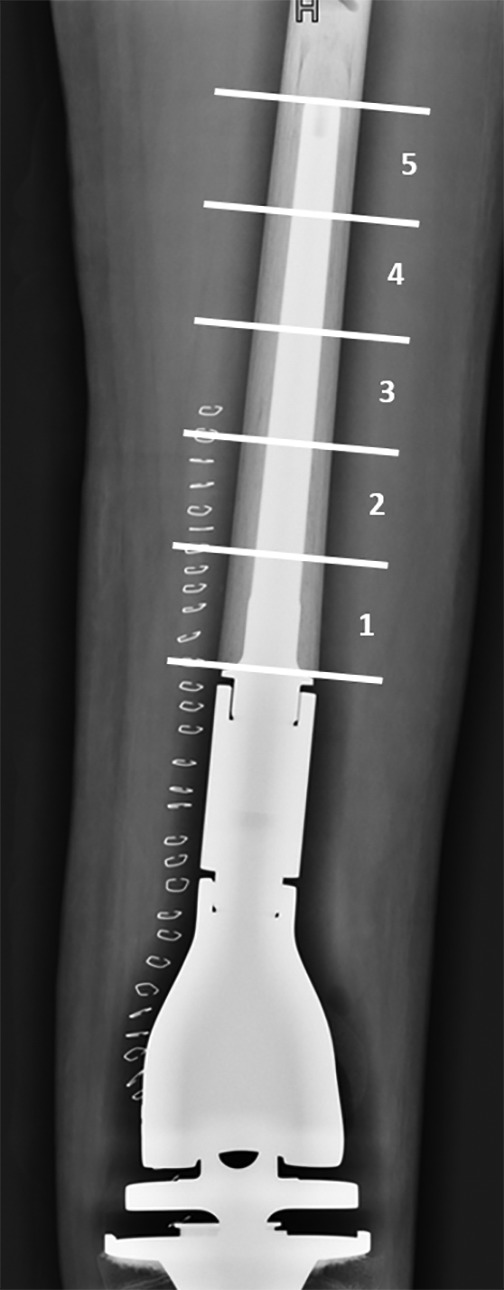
Radiographs of the stem of the distal femoral prosthesis (analysis zones).
